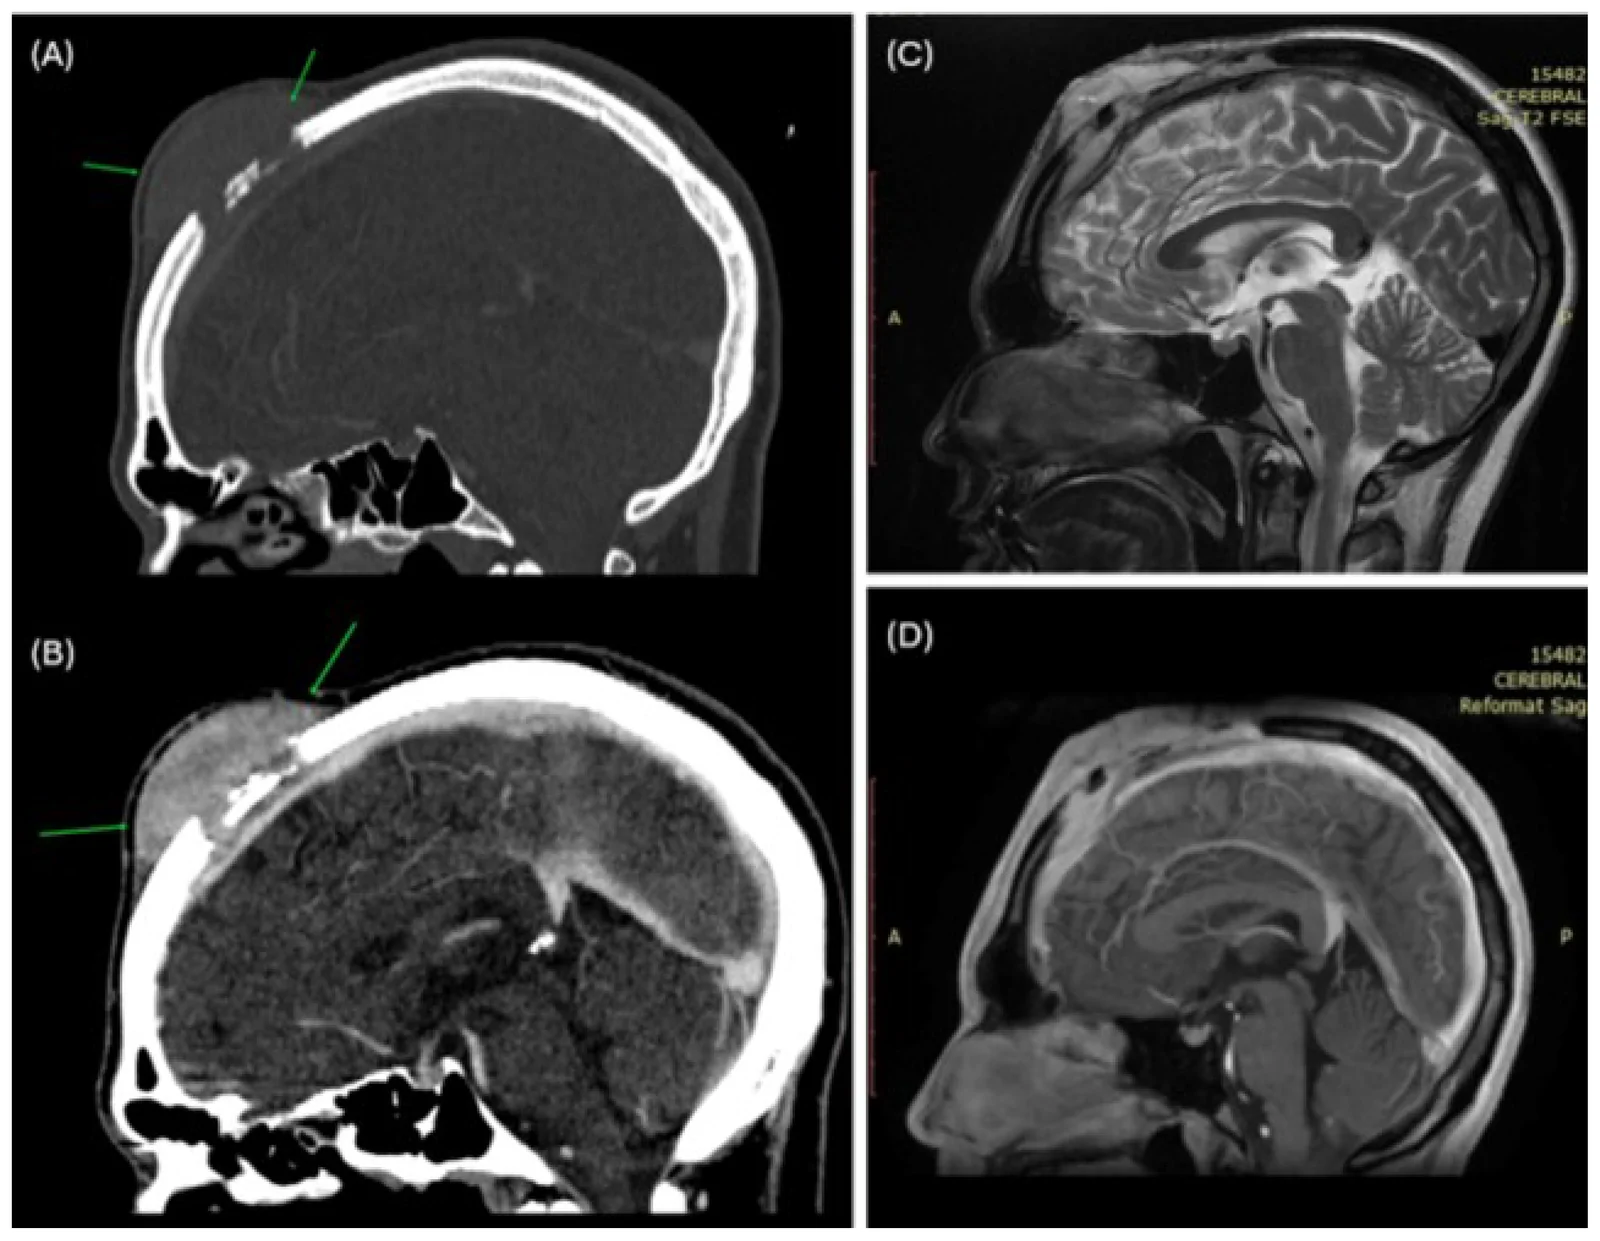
Angio-CT with venous time highlighted the frontal osteolytic tumor extended epicranially and invasive in the superior sagittal dural sinus (A,B). Postoperative brain MRI showed the dimensional increase in the frontal bone tumor (C,D). The letters A and P indicate anterior and posterior orientation.
